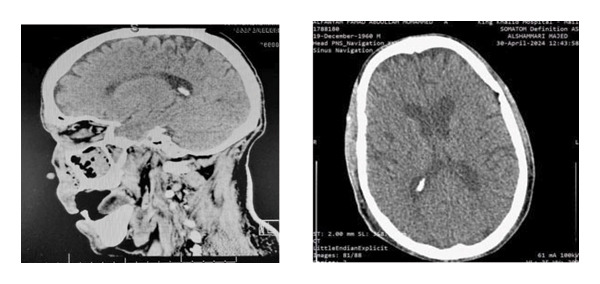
Sagittal CT scan demonstrating extensive opacification of the maxillary antrum and ethmoidal sinusitis.
